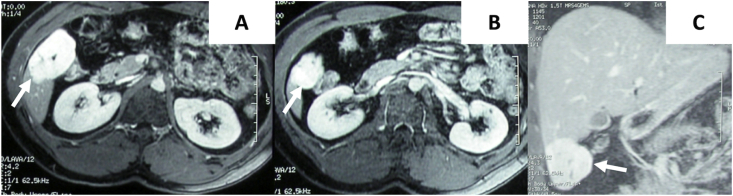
Axial gadolinium-enhanced MR images and Coronal gadolinium-enhanced MR scan of the hemangioma.
